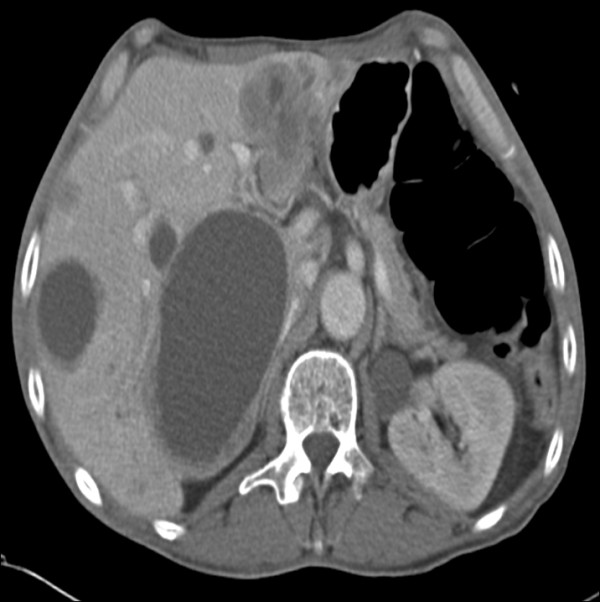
Distant metastasis-axial upper abdominal CT showing multiple tumour deposits and cysts in the liver.
